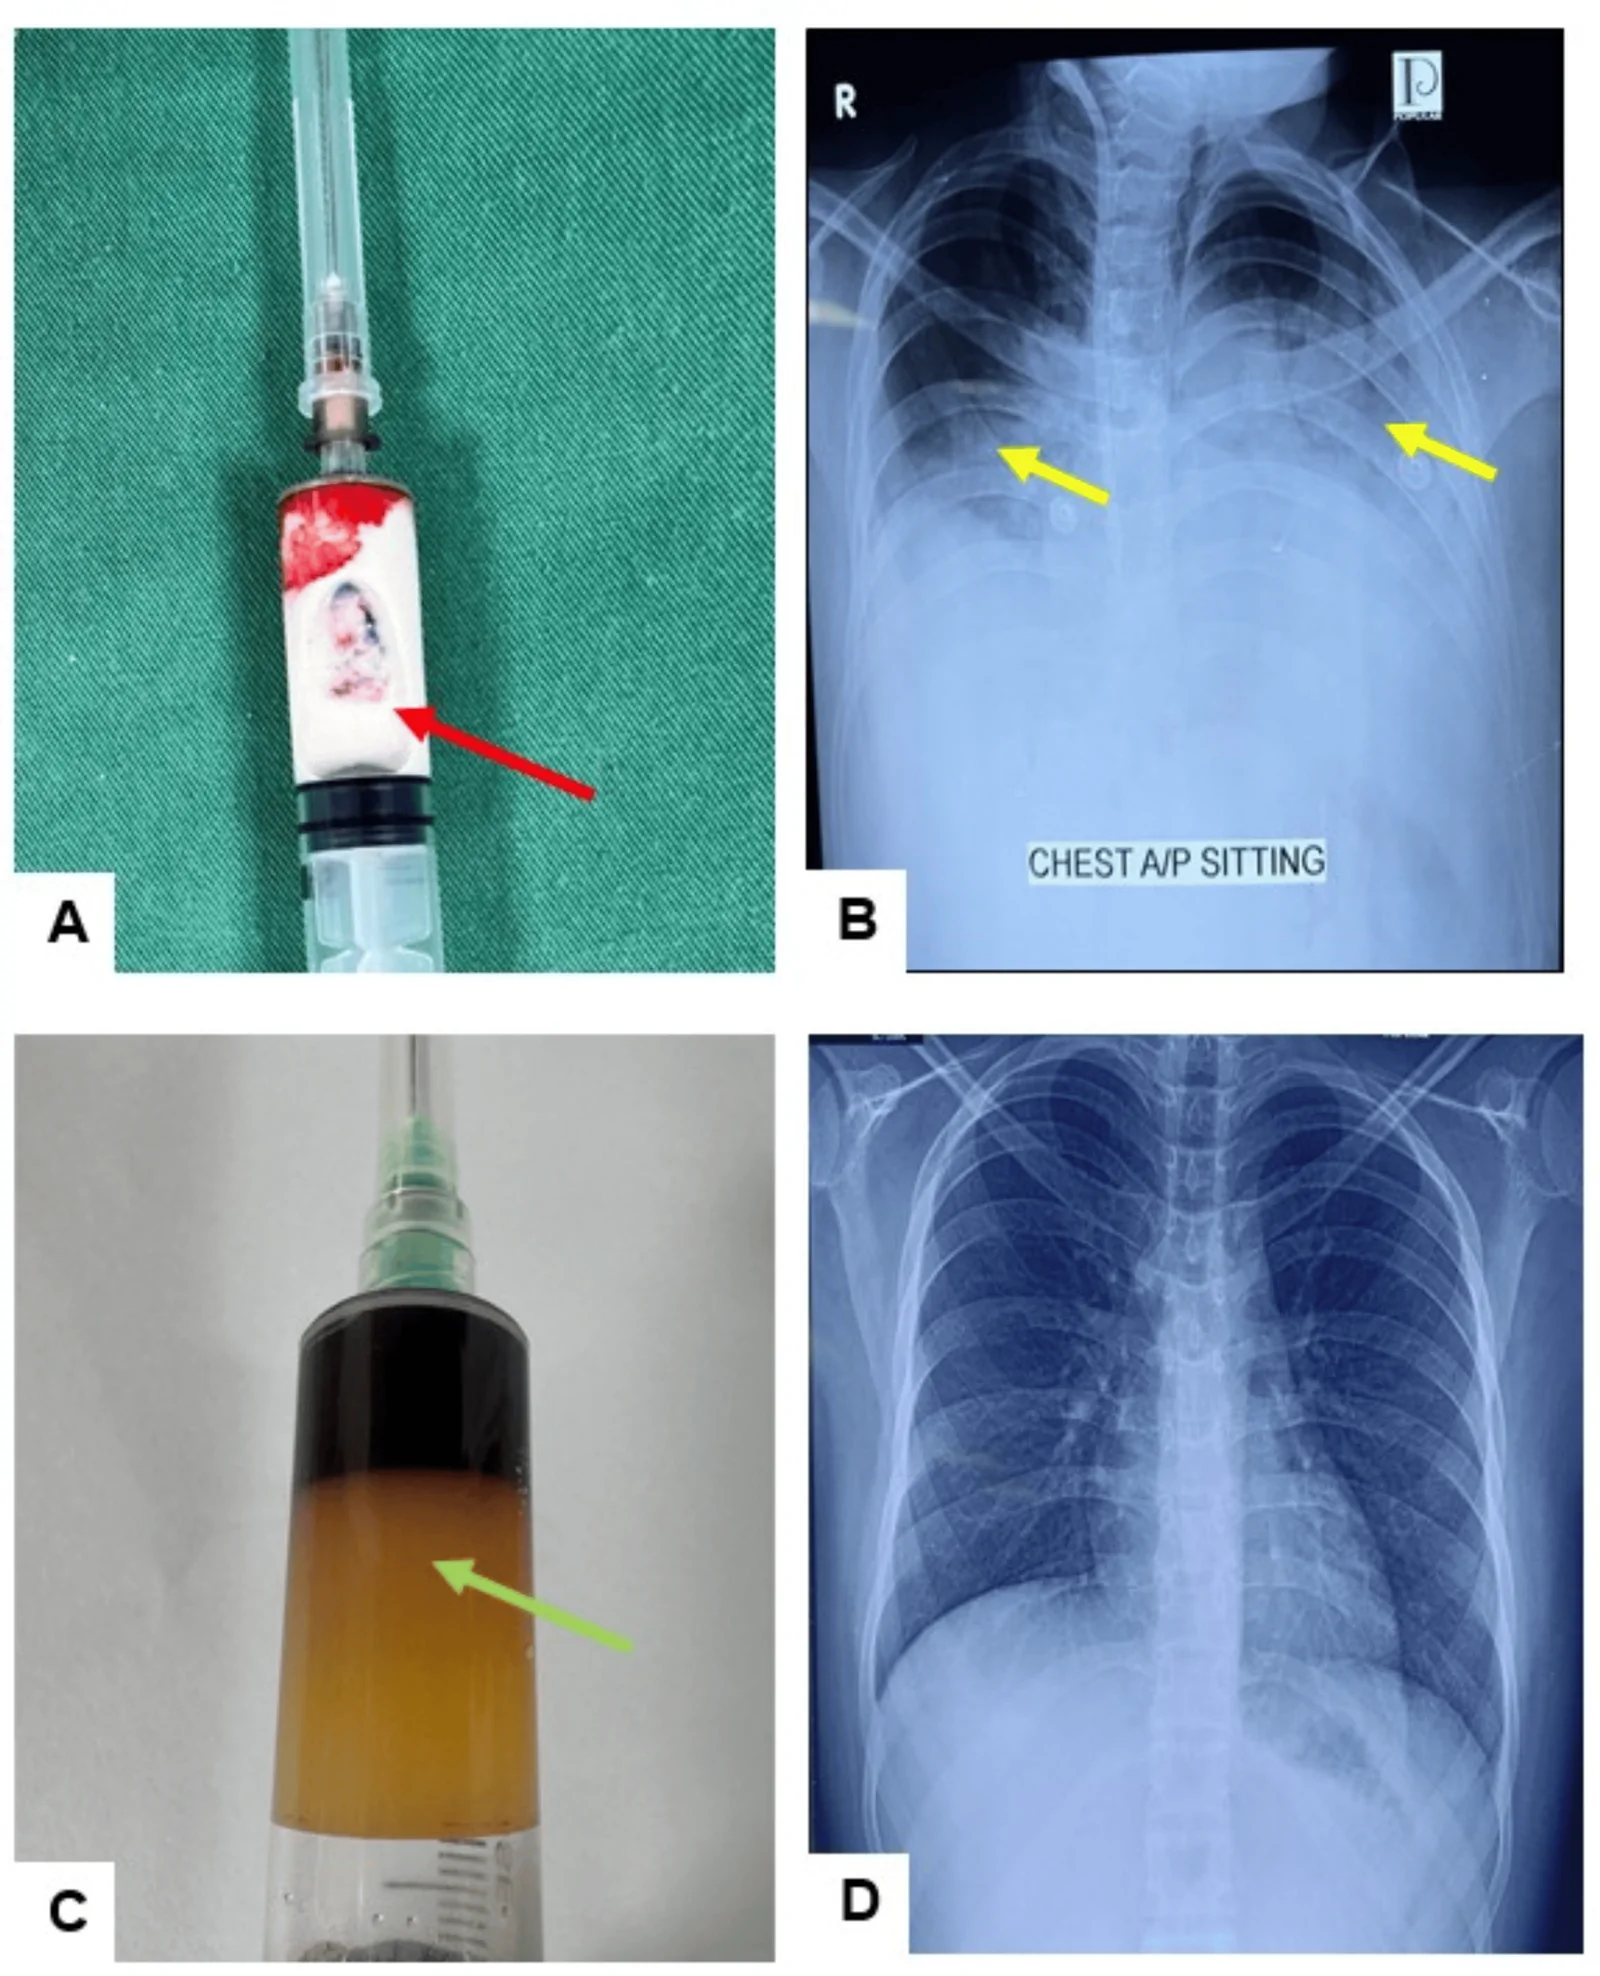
Clinical and radiographic findings before and after plasmapheresis (A) shows the patient's serum on the day of admission; the red arrow marks the white, thick, lipemic serum. (B) shows the admission chest radiograph with bilateral pulmonary Infiltrations (yellow arrow). (C) shows her serum after plasmapheresis (green arrow). (D) almost normal chest radiograph just before discharge
